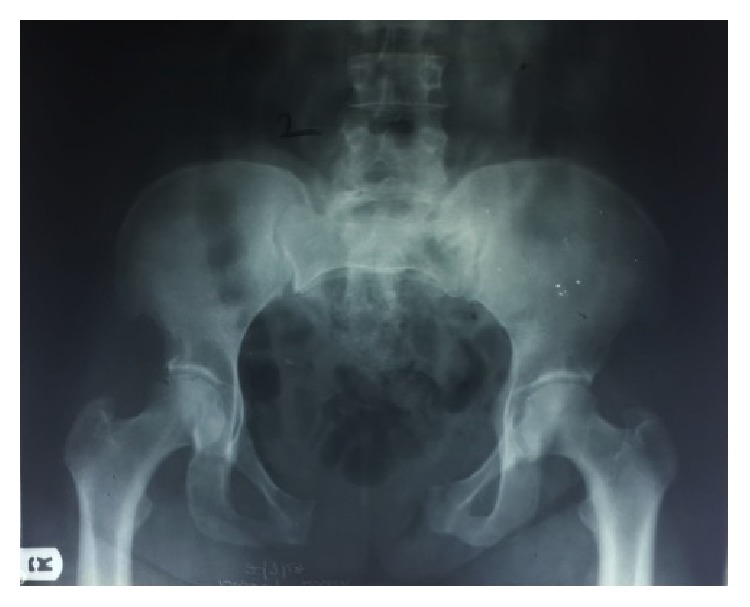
Pelvic X-Ray showing wide pubic diastasis, fractured left upper ischial ramus, and widened sacroiliac joints.
